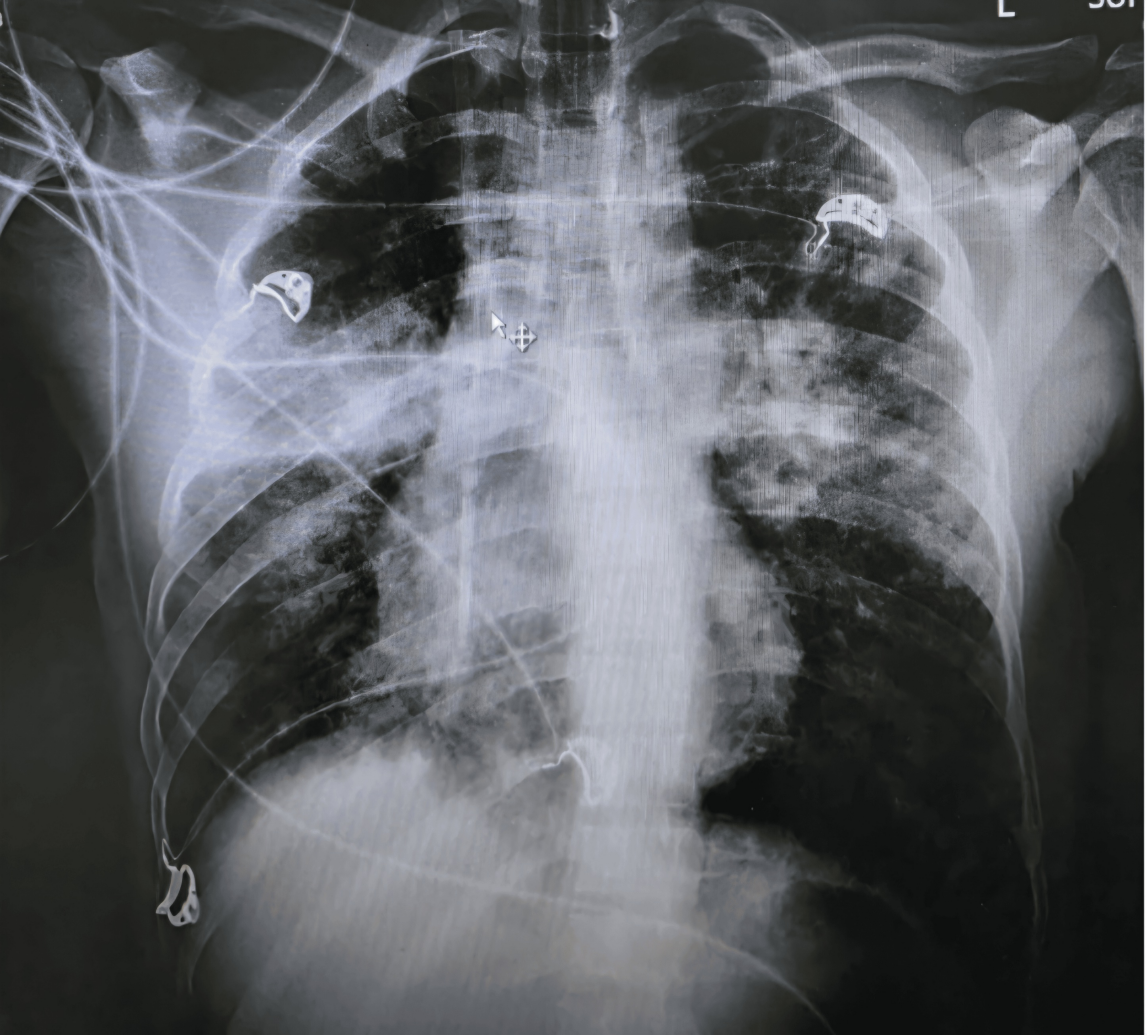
Chest radiograph showing fluid overload pulmonary edema and pleural effusion one day after admission
